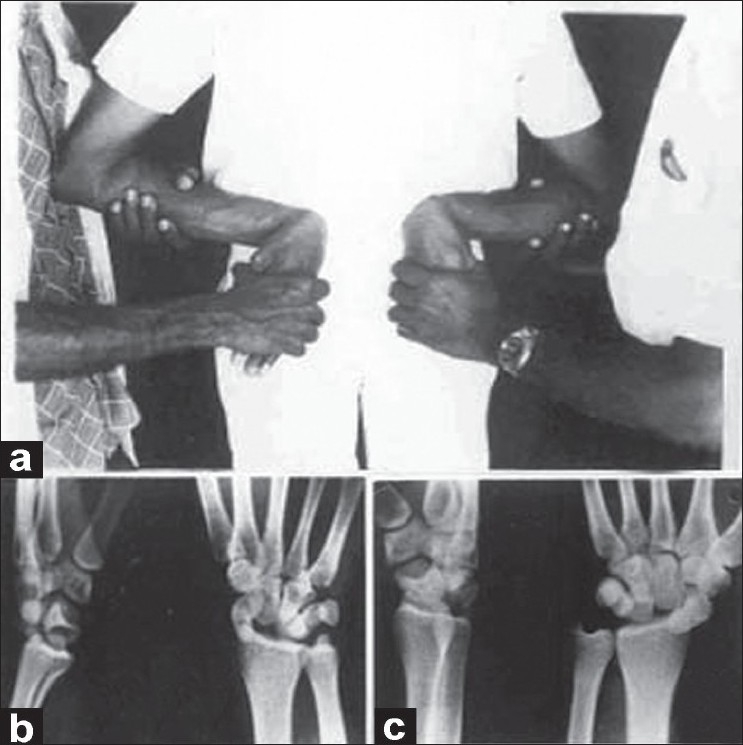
(a) Clinical photograph showing the position of limbs held on back in forcible full volar flexion, radial deviation and pronation at the wrists.(b) Lateral and anteroposterior X-rays of left and right.(c) wrists showing posterior perilunate dislocation. Note: Irregularity of posterior horn of lunate in lateral view
